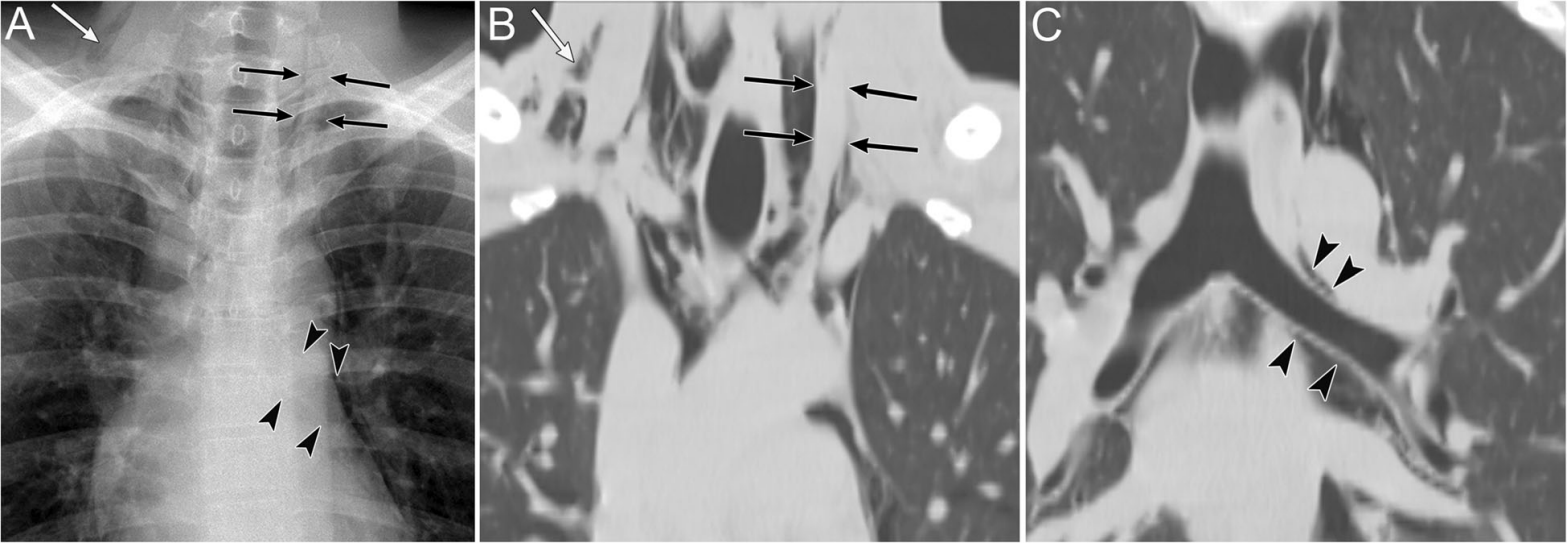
Pneumomediastinum due to chronic cough and vomiting in a 22-year-old man. a A supine radiograph shows the “tubular artery” sign (black arrows) and the “double bronchial wall” sign (black arrowheads). Subcutaneous emphysema is also evident (white arrow). b A coronal cervical CT image shows the “tubular artery” sign (black arrows). Both sides of the left internal jugular vein are visible due to pneumomediastinum. Subcutaneous emphysema is also visible (white arrow). c The coronal CT image at the carina level shows the “double bronchial wall” sign (black arrowheads). Both sides of the left bronchial wall are visible because of air in the bronchus and pneumomediastinum surrounding the wall
